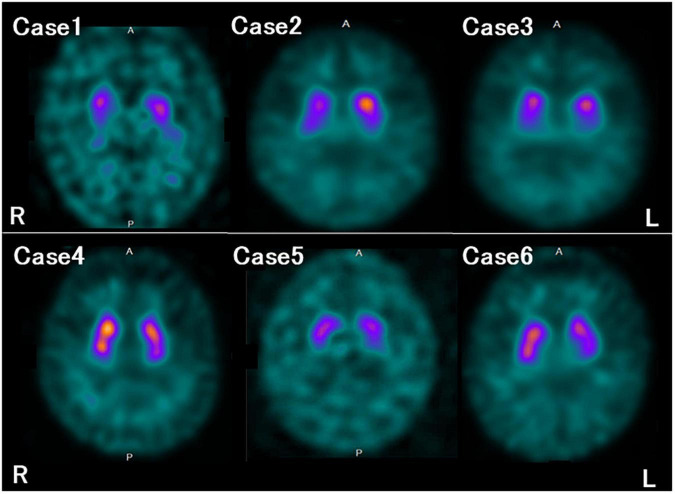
Dopamine transporter (DAT) single photon emission computed tomography (SPECT) findings in each case. All the patients in this study showed reduced DAT availability.
